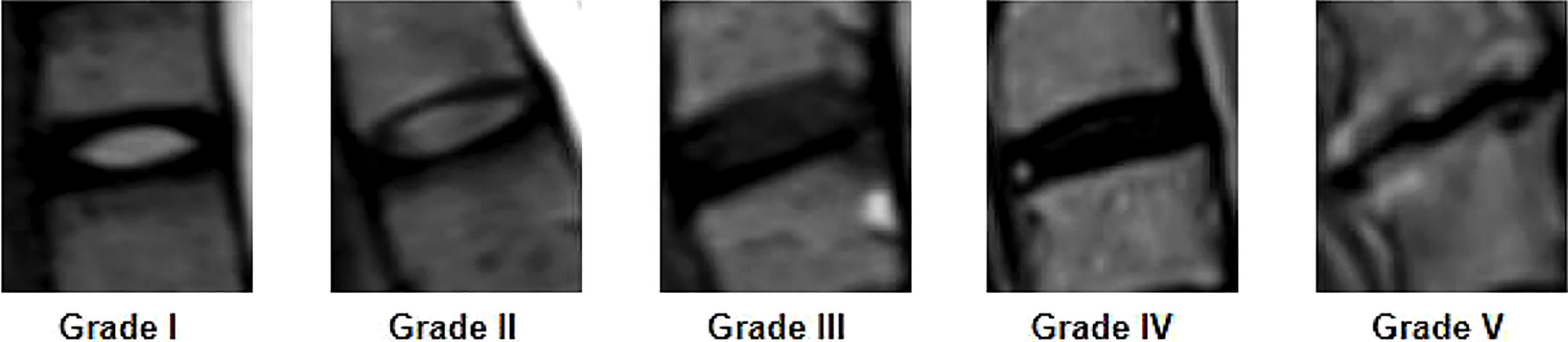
Cervical disc rating on MRI by using the Pfirrmann classification from grade I to grade V.
